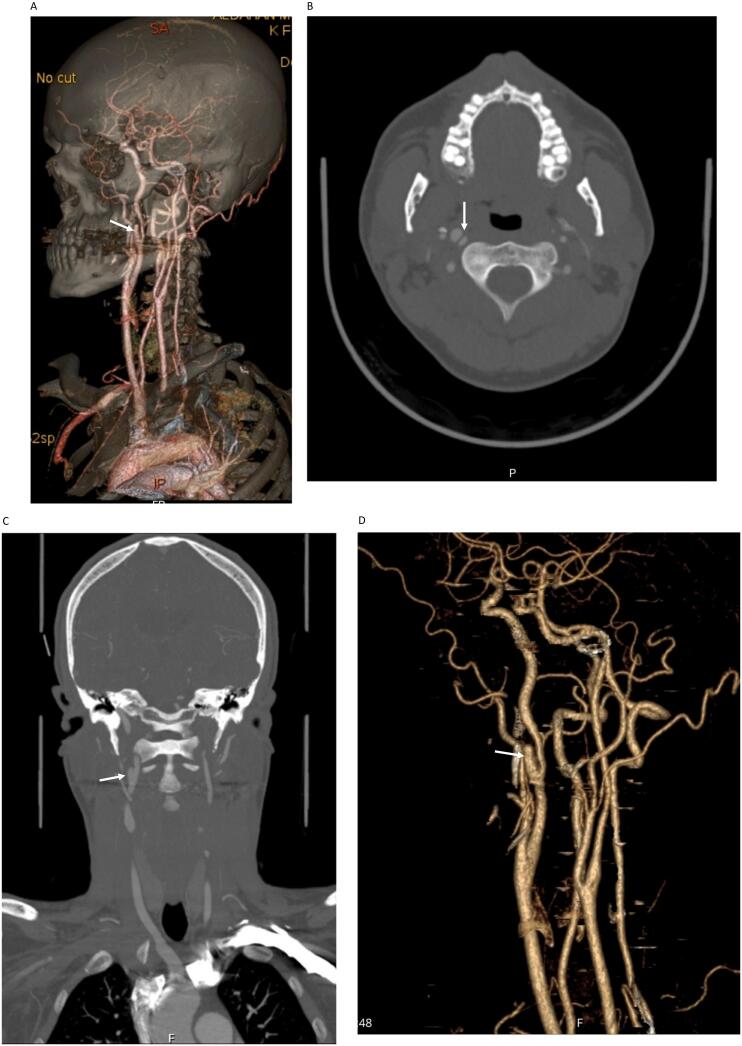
A: 3D volume rendering showing right ICA pseudoaneurysm (white arrow). B: CT angiography axial section showing no left ICA dissection, and evident right ICA dissection and pseudoaneurysm (white arrow). C: CT angiography coronal reconstruction showing right ICA pseudoaneurysm (white arrow). D: VR 3D reconstruction showing ICA pseudoaneurysm (arrow).
